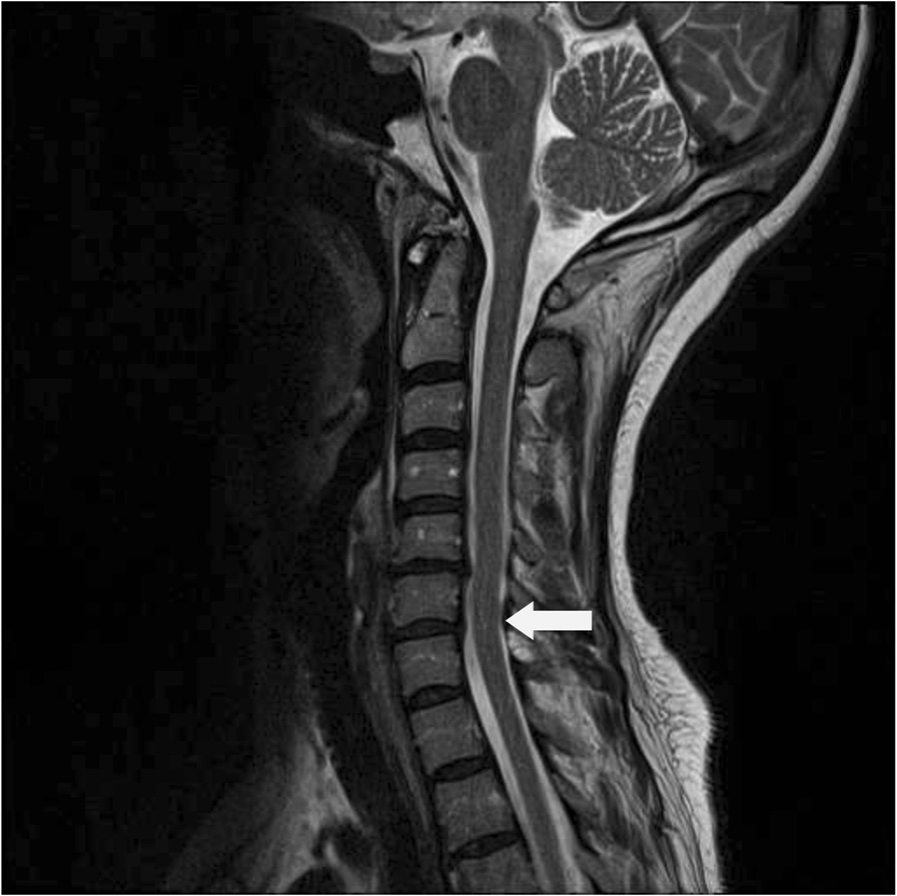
MRI T2 weighted sequence of the cervical MRI after Prednisone, Cyclophosphamide and Mycophenolate therapy, demonstrates improvement and near resolution of previously noted signal abnormalities.
